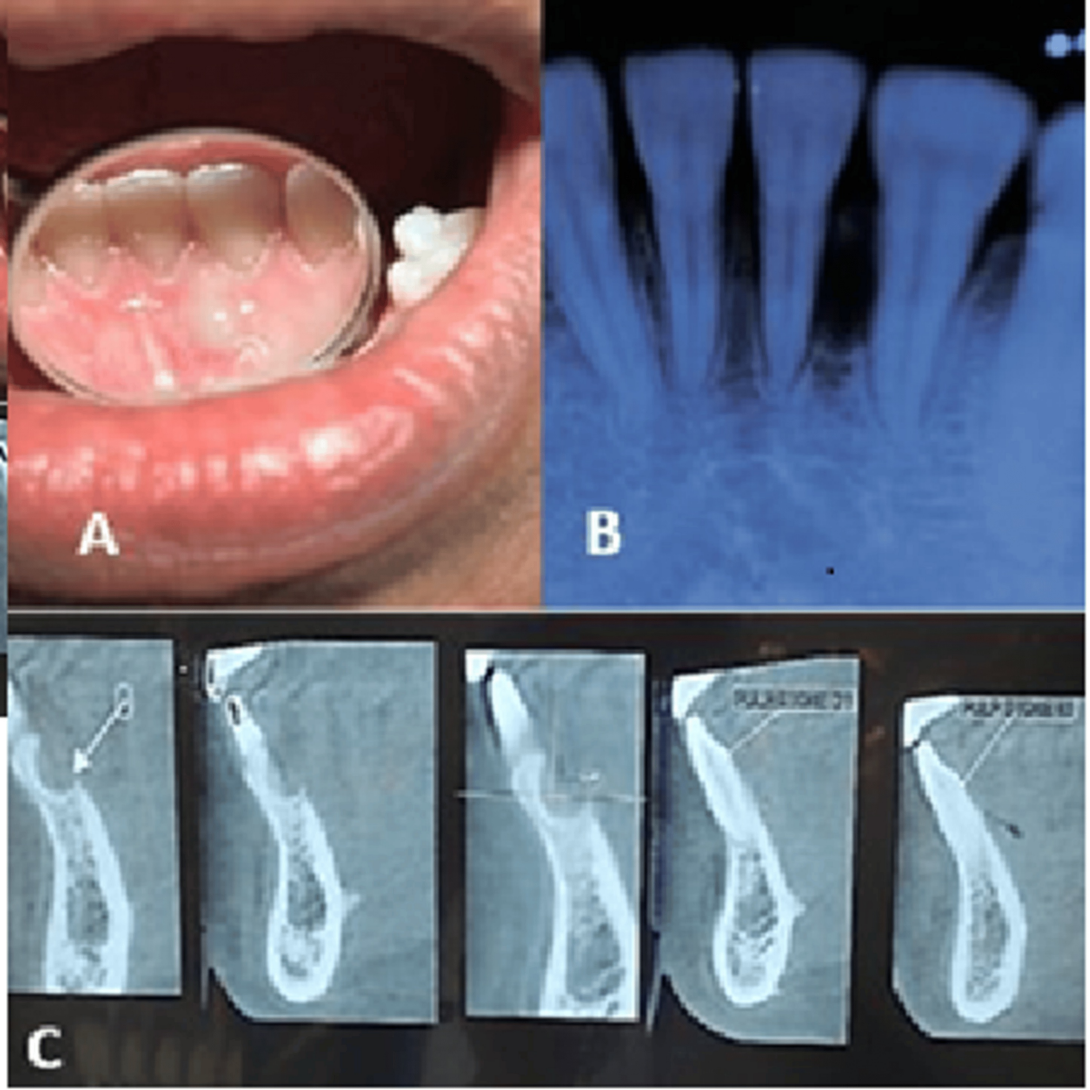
(A) Preoperative view of the swelling from the lingual aspect. (B) Preoperative intraoral periapical radiograph of the teeth 31 and 32 region. (C) Cone-beam computed tomography view showing thinning and perforation of the lingual cortical bone.
